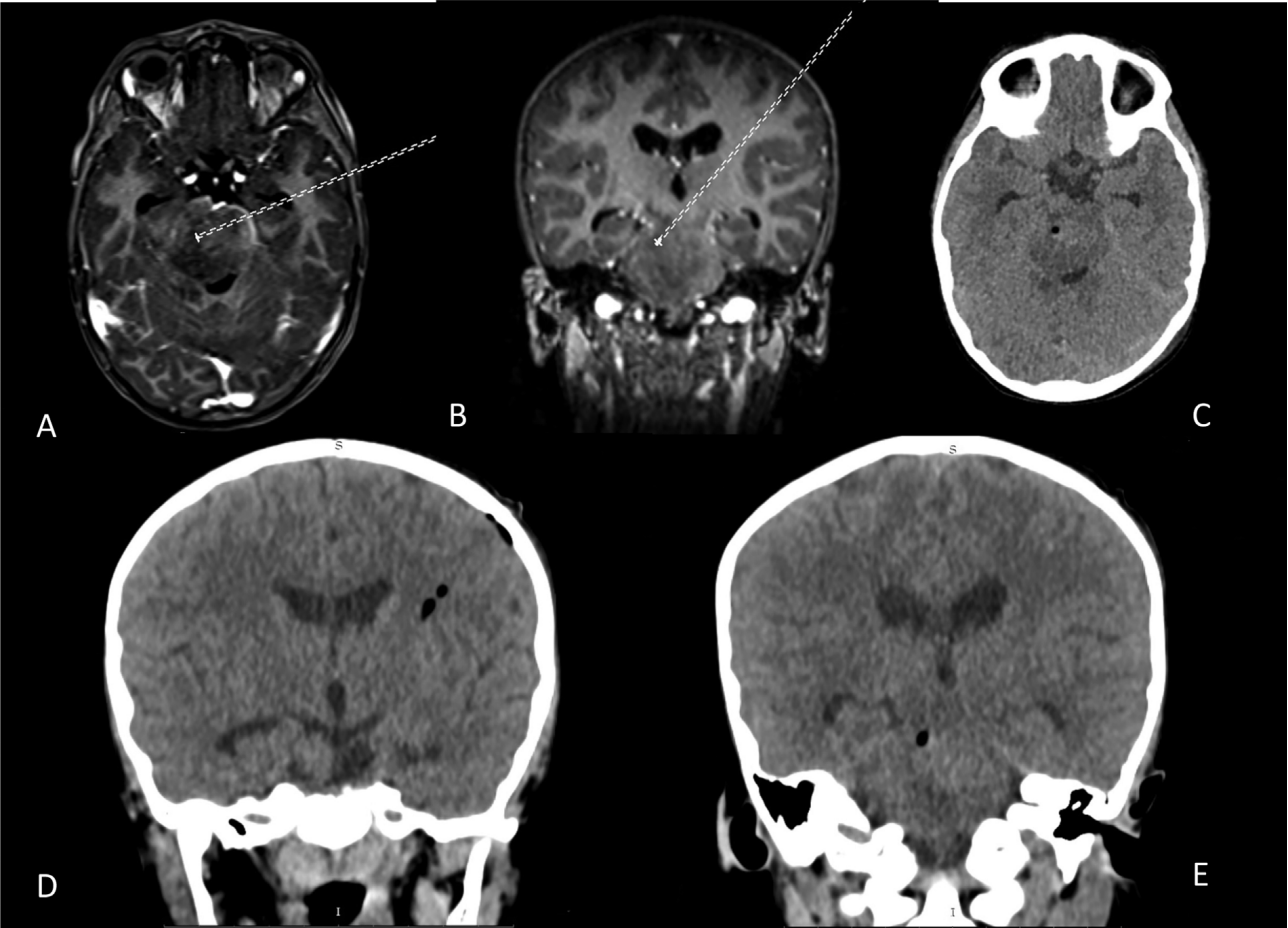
Preoperative brain magnetic resonance imaging axial view (
